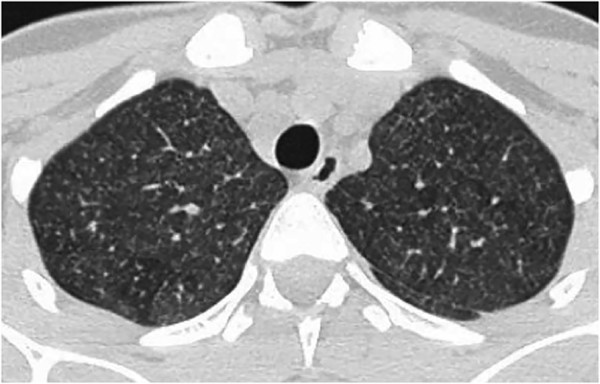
Axial CT scan of the upper lobes of the lung showed numerous, bilateral, poorly defined and randomly distributed small pulmonary nodules with a preferentially centrilobular location.
